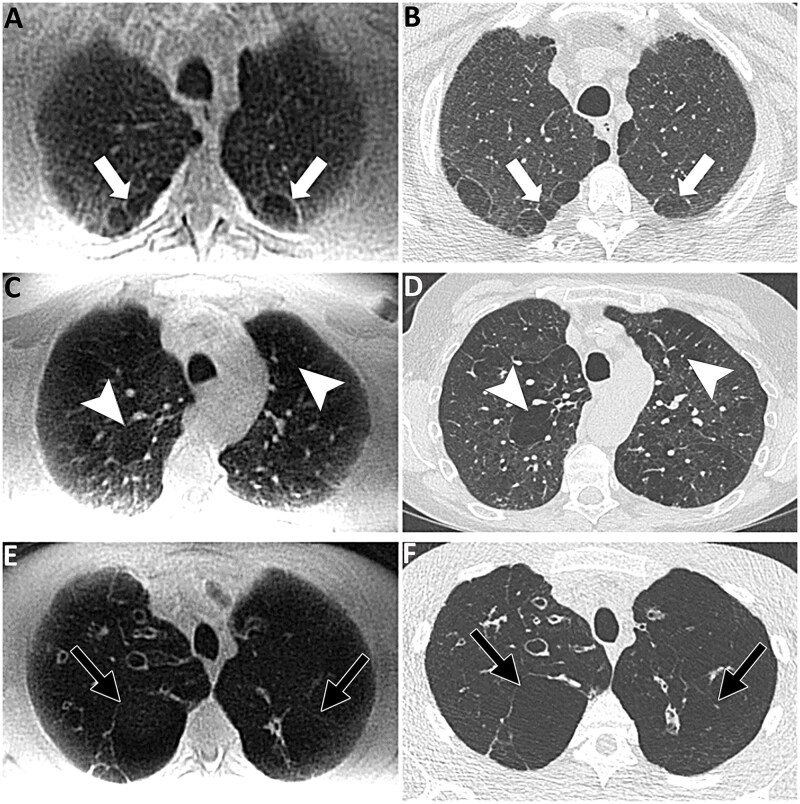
Three-dimensional images obtained at, A, C, E, ultrashort echo time MRI and, B, D, F, CT in 3 study participants with chronic obstructive pulmonary disease. A, B, Images in the first participant show mild paraseptal emphysema (arrows). C, D, Images in the second participant show moderate emphysema (arrowheads indicate areas of centrilobular emphysema). E, F, Images in the third participant show severe panlobular emphysema; there are conspicuous fine septal lines delineating hyperinflated lobules (arrows). Reproduced with permission from Benlala I, et al. Radiology, 2019, Jul;292(1):216-225. © 2019 Radiological Society of North America.^27^
